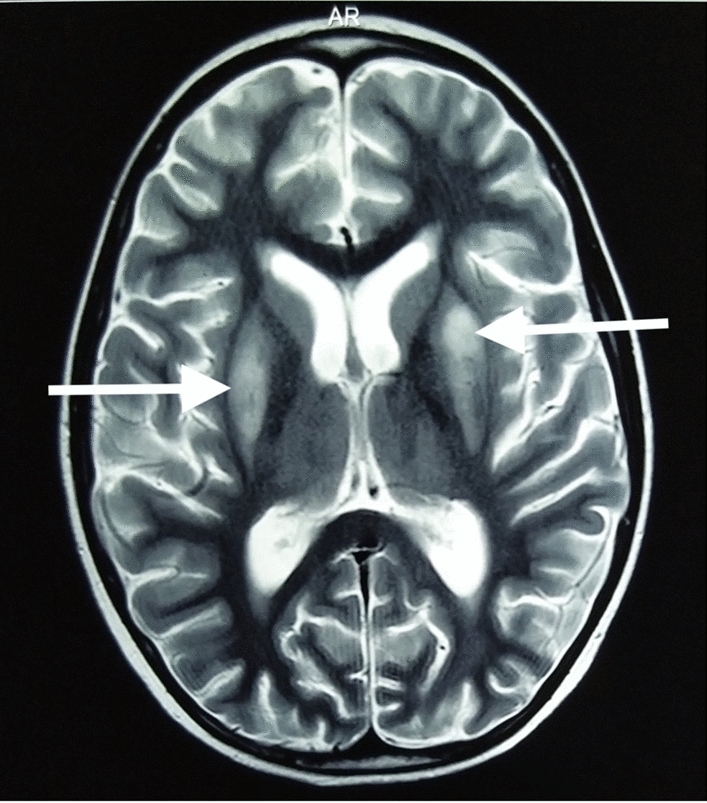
Brain MRI showing almost symmetrical T2 high signal intensities with diffusion restriction of bilateral putamen (arrow) and subcortical areas and deep white matter
